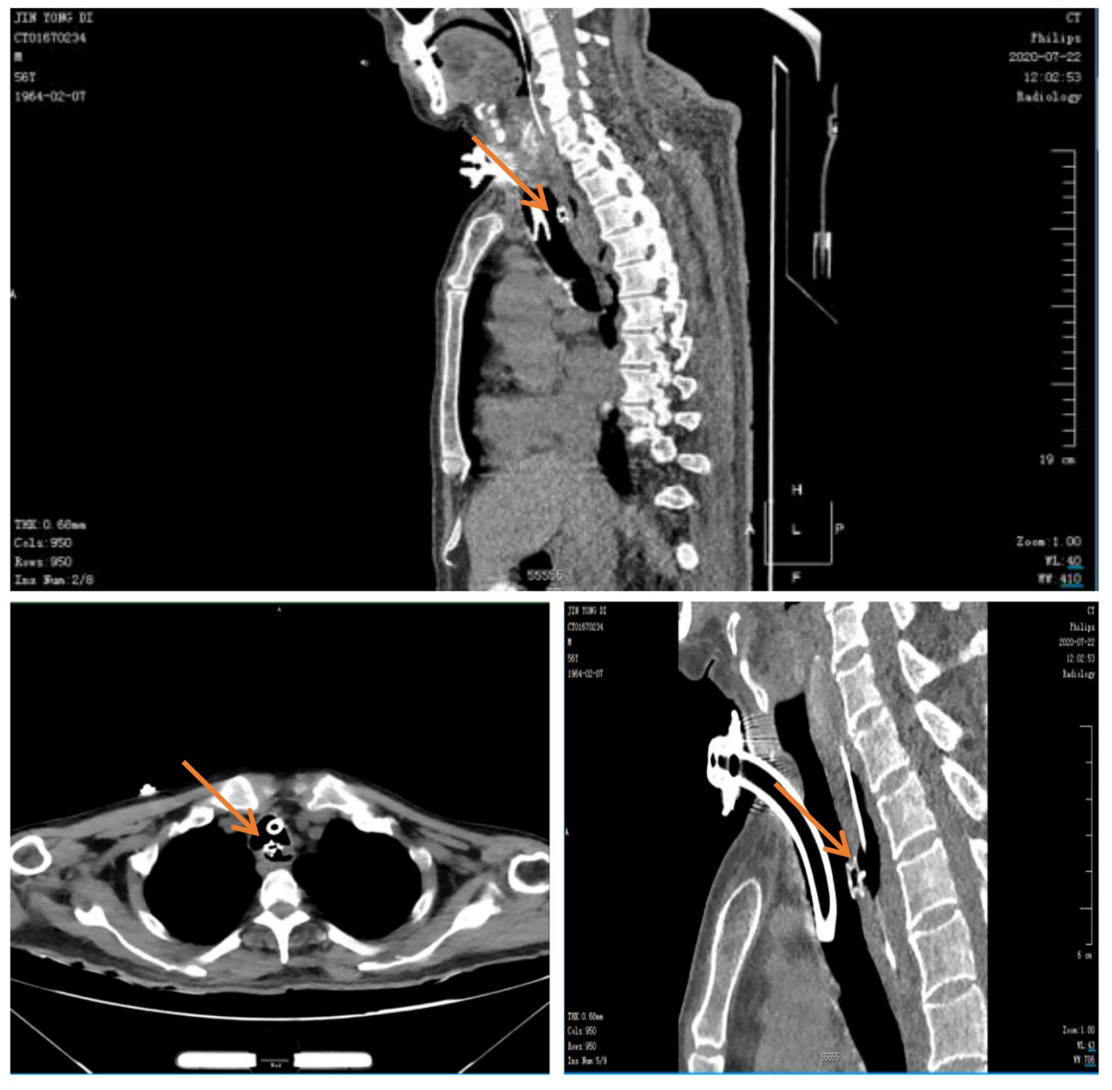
Transverse and sagittal views of occluder position on postoperative CT. The location of the occlusion device is indicated by the arrow
